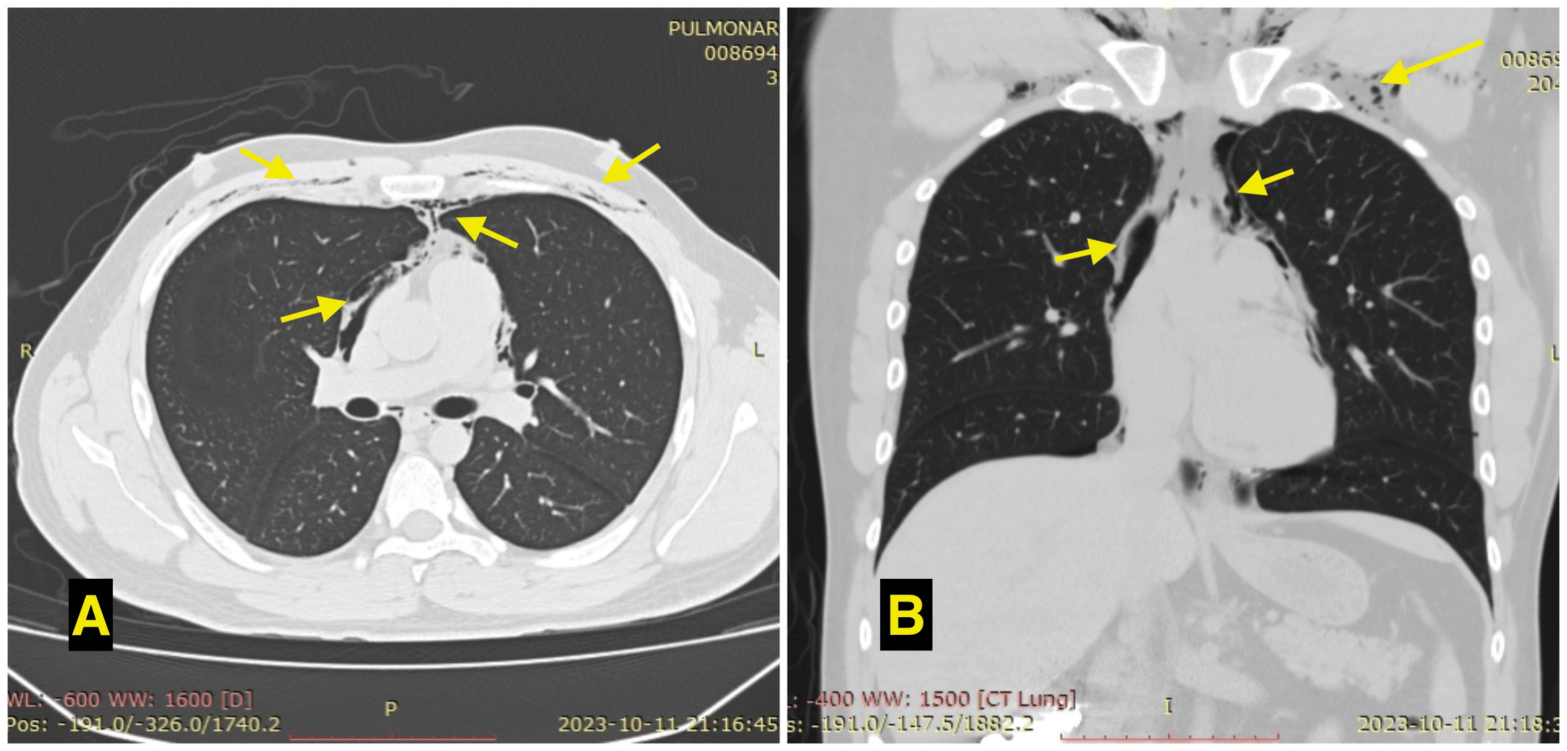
A: CT scan in axial view; the arrows point to pneumomediastinum and subcutaneous emphysema. B: CT scan in coronal view; the arrows point to pneumomediastinum and subcutaneous emphysema.
